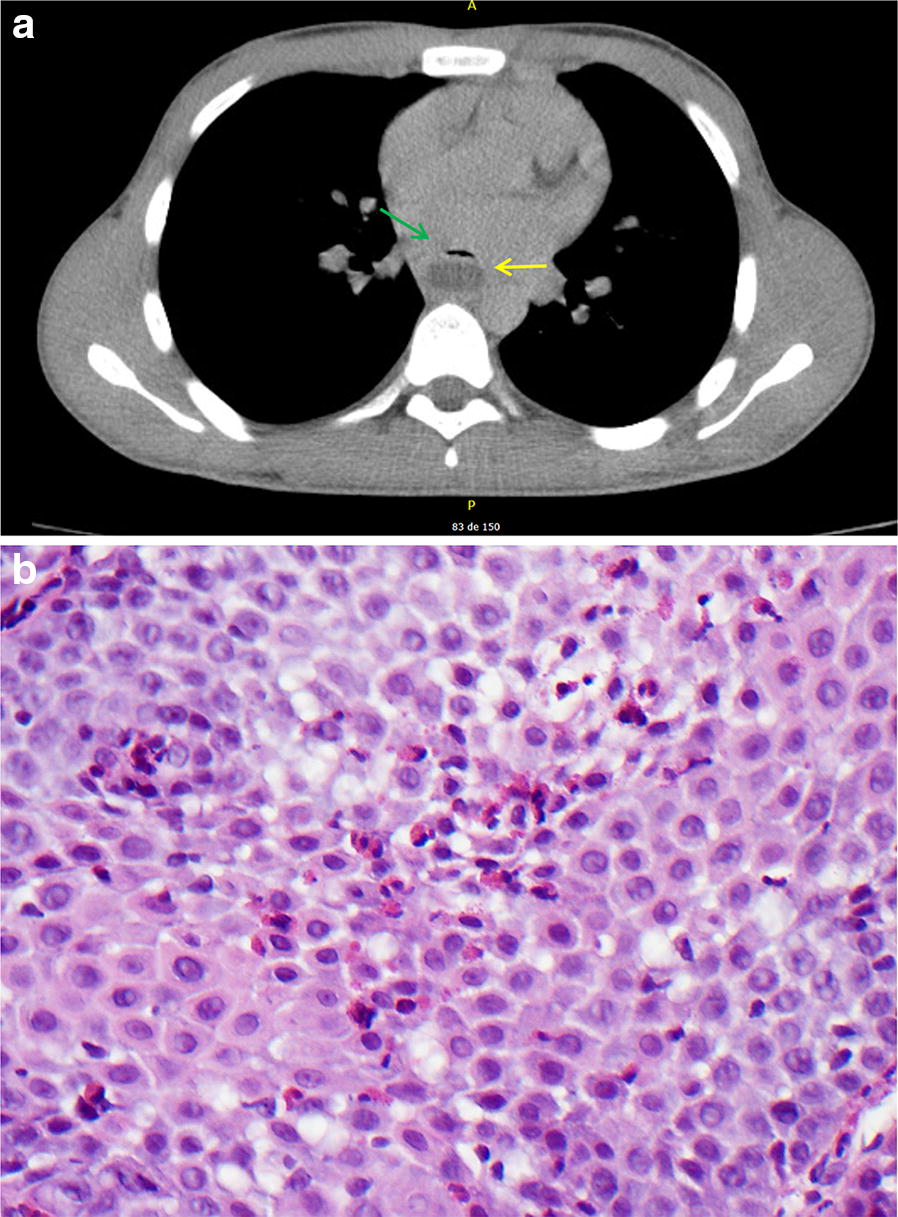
a CT image with the mediastinal abscess (yellow arrow) behind the esophagus (green arrow). b Pathological findings showing diffusely infiltrated eosinophils, with microabscess formation
